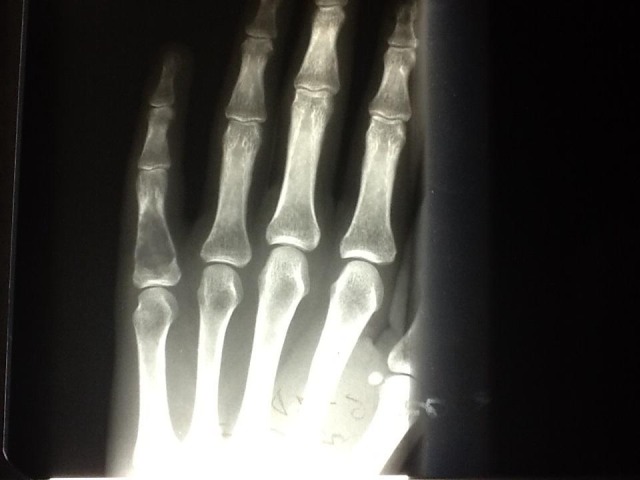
Frontal radiography of little finger
